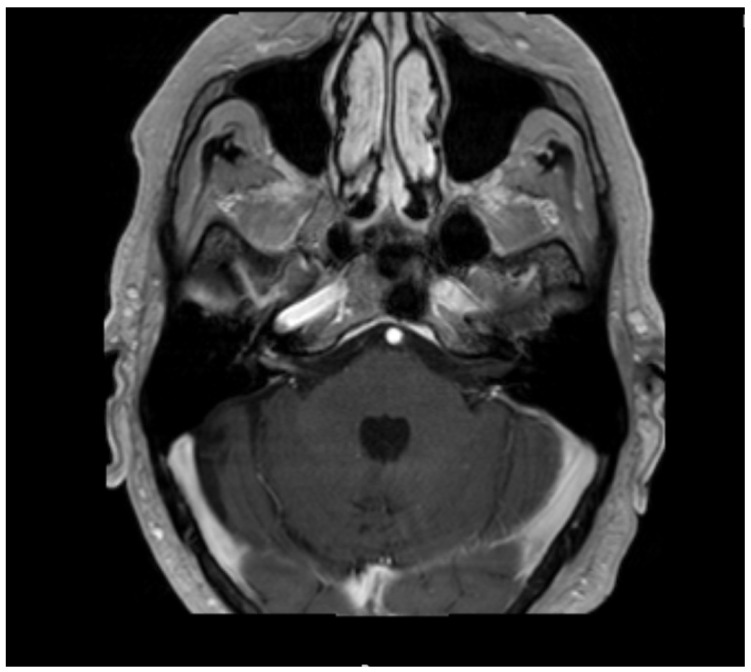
The MRI of brain showed linear enhancement of the 7th and 8th cranial nerves (CN) within the internal auditory canal, which is indicative of inflammation of the two cranial nerves.
